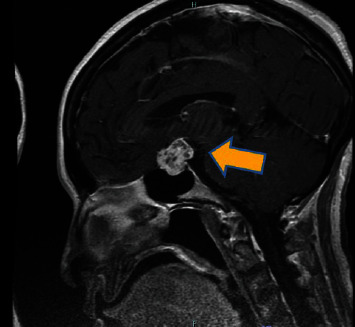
The sagittal T1-weighted image shows an inhomogeneous enhancing mass arising from the sella turcica, indicating a mass measuring 1.5 × 2.0 × 2.2 cm.
